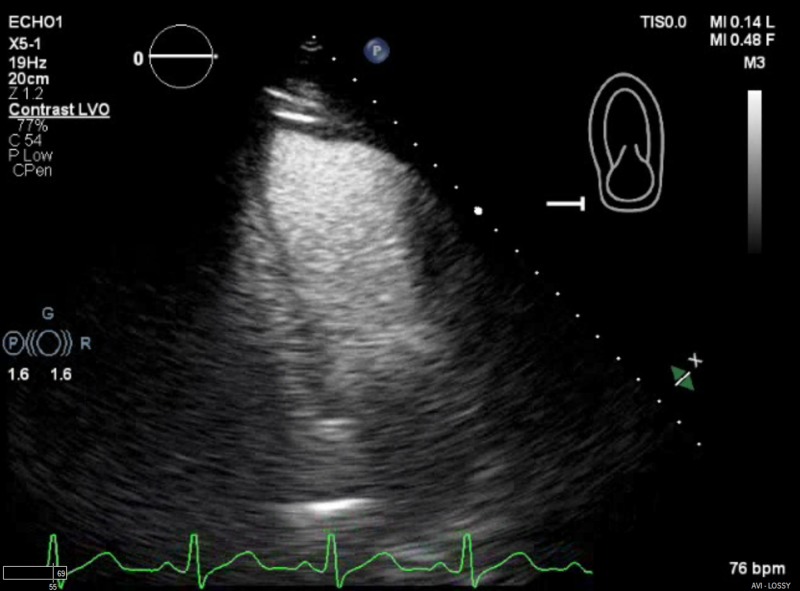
Echocardiogram Severe anterolateral and basal hypokinesia
